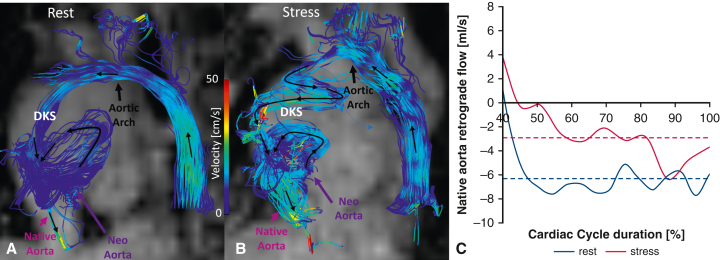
A and B, Four-dimensional flow MRI streamlines of retrograde flow from the descending aorta into the native aorta at rest and stress, respectively. C, Diastolic retrograde flow: consistently with the flow dynamics shown in (A) and (B), the retrograde flow rate is higher at rest than at stress, with *dashed lines* indicating mean flow rates (6.1 mL/s vs 3 mL/s). *MRI*, Magnetic resonance imaging.
